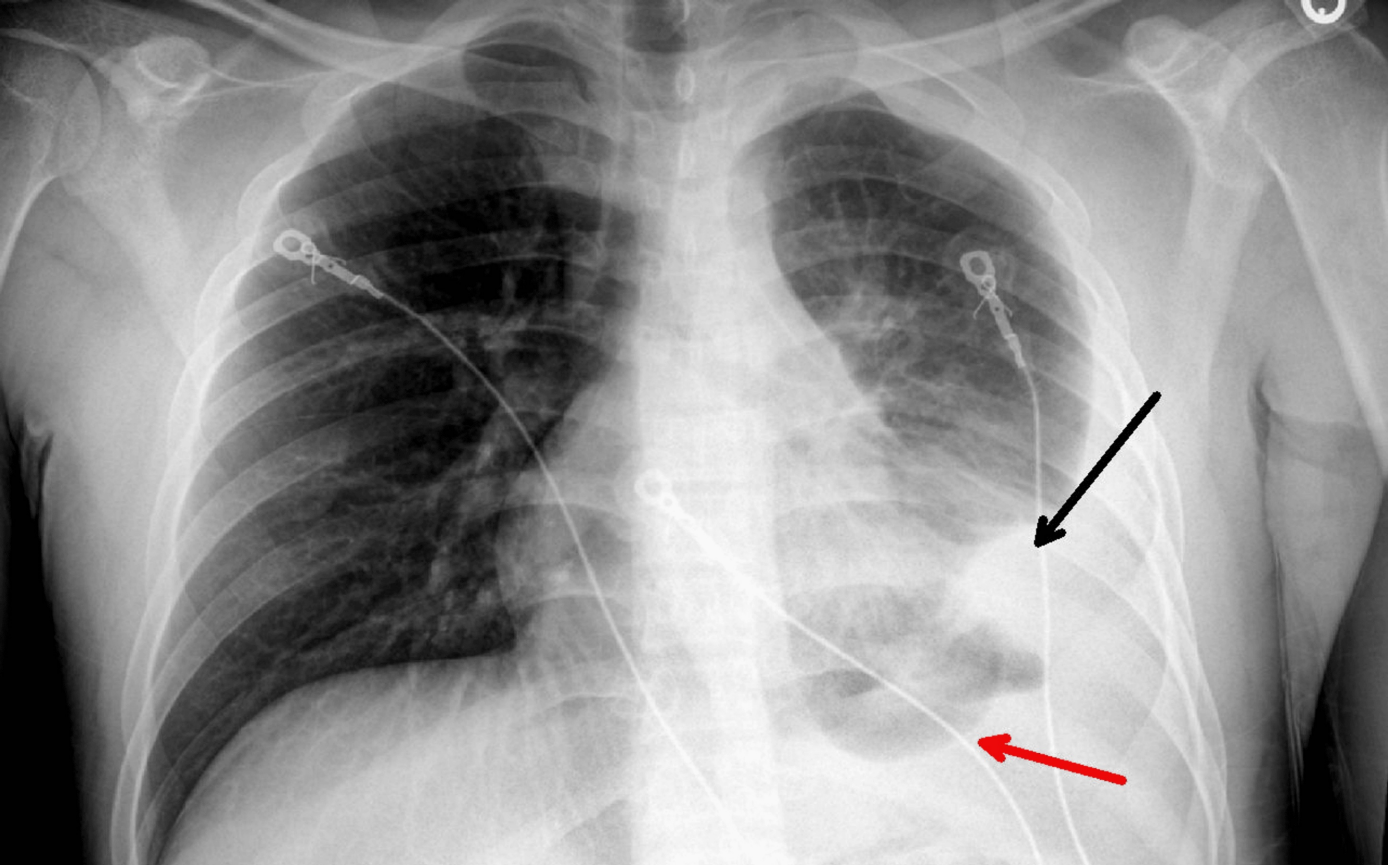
Chest X-Ray showing atelectasis (black arrow) and pleural effusion (red arrow) in the left lung.
